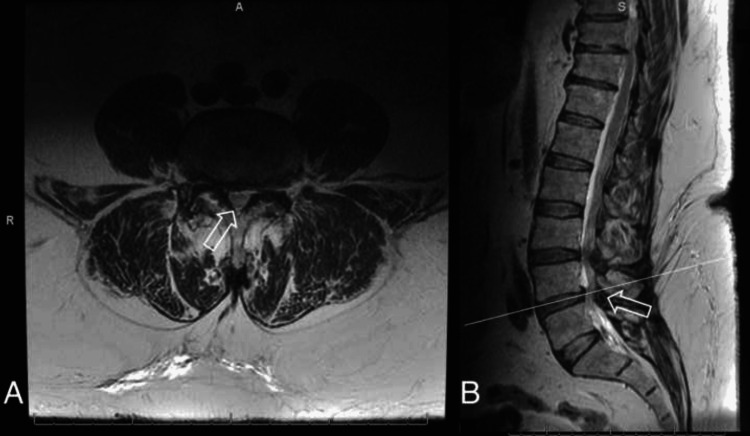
(A) Axial and (B) sagittal T2-weighted MRI of the lumbar spine demonstrating severe spinal stenosis at L4-L5 (arrows).
